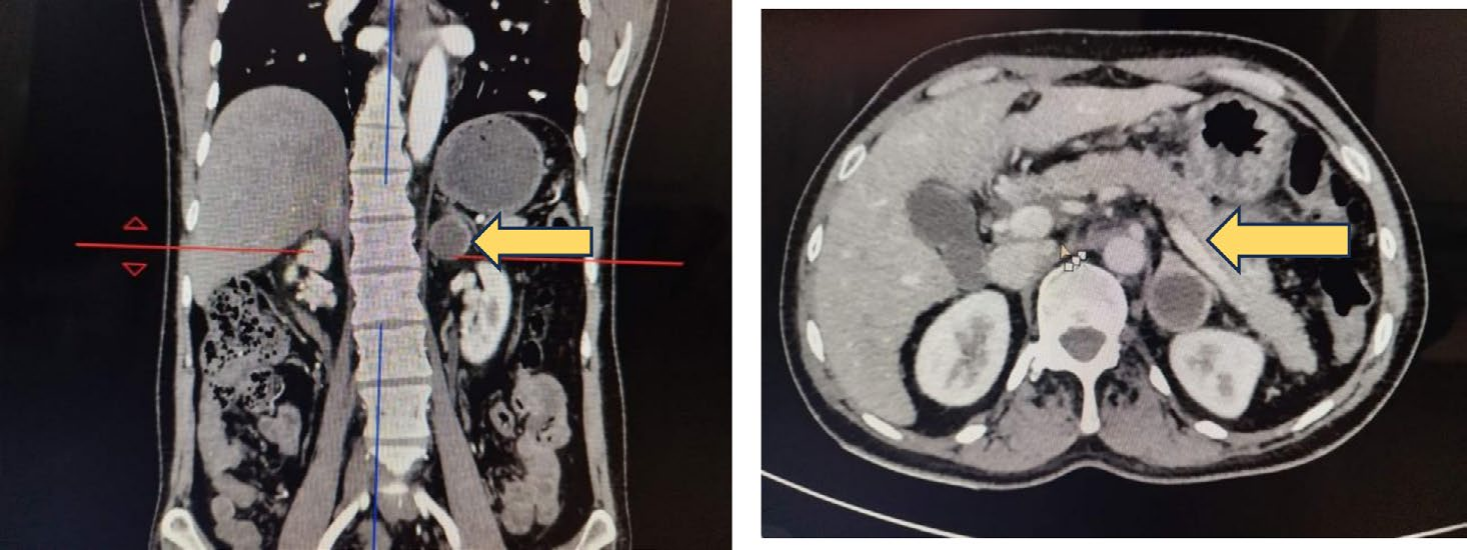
Follow‐up contrast enhanced computed tomography (CECT) of abdomen and pelvis of index case showed left adrenal lesion measuring 28 mm × 27 mm × 26.3 mm (yellow arrowhead).
